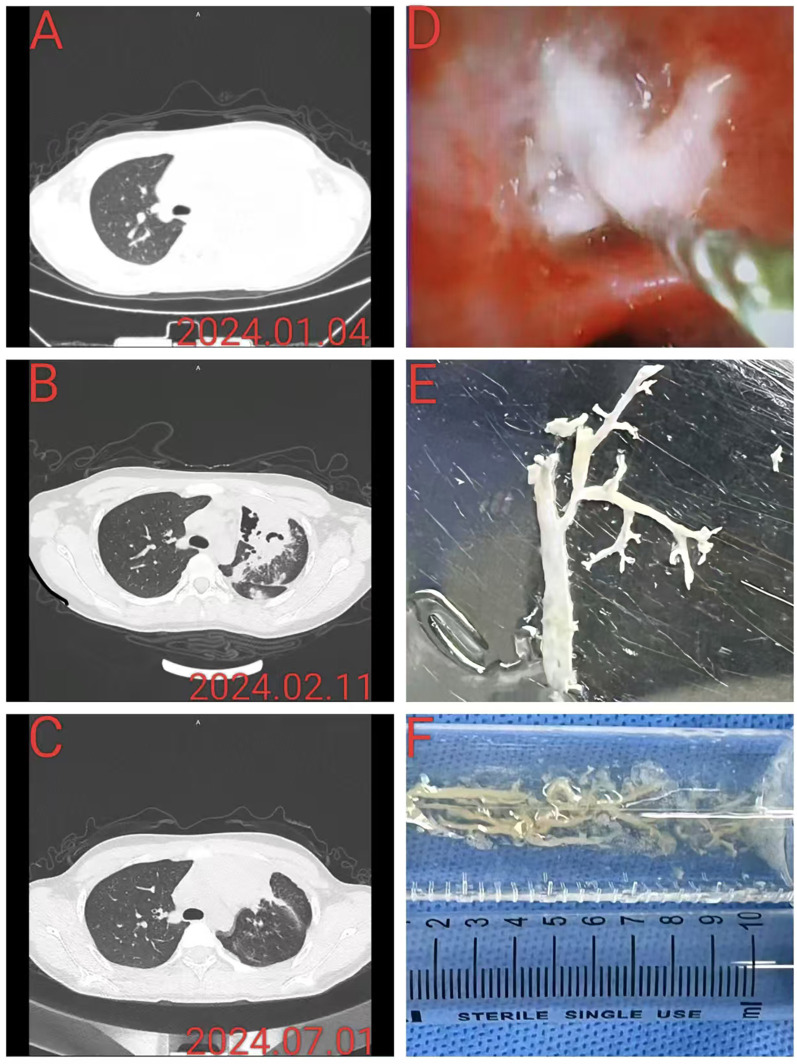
This patient had severe *Mycoplasma pneumoniae* pneumonia complicated by plastic bronchitis. (A) A chest CT at admission revealed left upper lobe consolidation. (B) Chest CT reexamination approximately 1 month after discharge revealed partial pulmonary necrosis in the left upper lobe, accompanied by bronchopneumonia. (C) Chest CT re-examination approximately 6 months after discharge revealed localized atelectasis in the left upper lobe, accompanied by slight chronic inflammation. (D) Under bronchoscopy, white rubber-like sputum plug blockage and foreign body forceps removal were performed. (E,F) The plastic object with a tree-like shape was removed and placed in a saline tray, and the longest branch was approximately 7 cm long.
